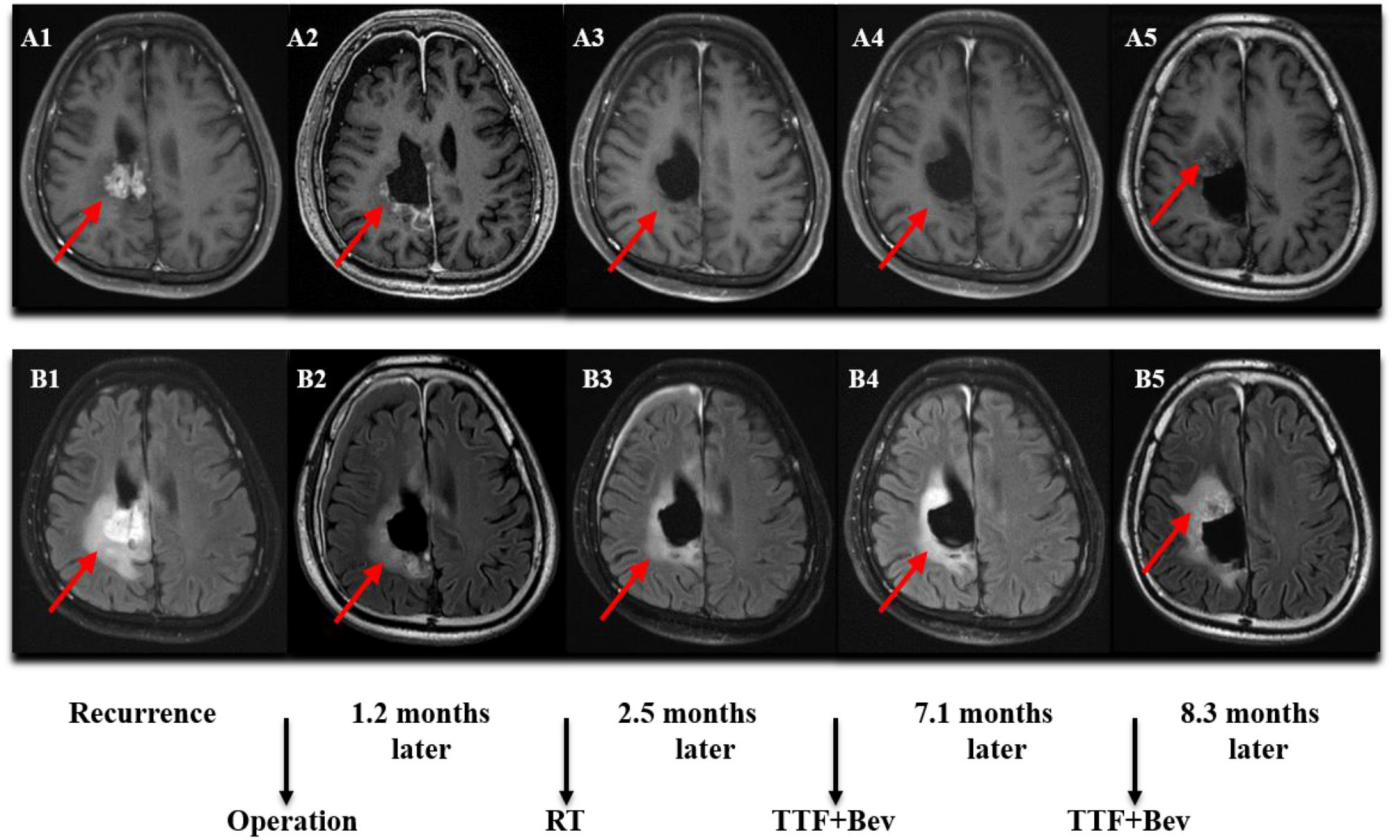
MRI (Magnetic Resonance Imaging) changes of recurrent GBM (rGBM). (A1–A5) Contrast-enhanced MRI; (B1–B5) MRI-Flair. MRI images followed-up every 2 months before and after treatment. Only some of the images were exhibited. The arrow indicates the tumor and tumor bed. This patient was followed-up for 8.3 months and he developed a disease progression.
